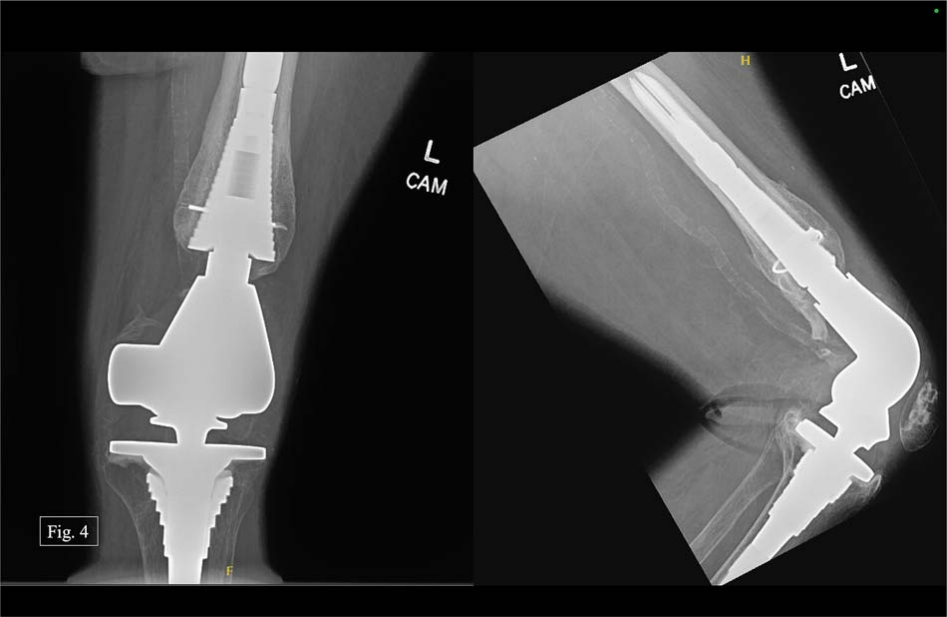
Left, 48 month postoperative AP and lateral X-rays.
